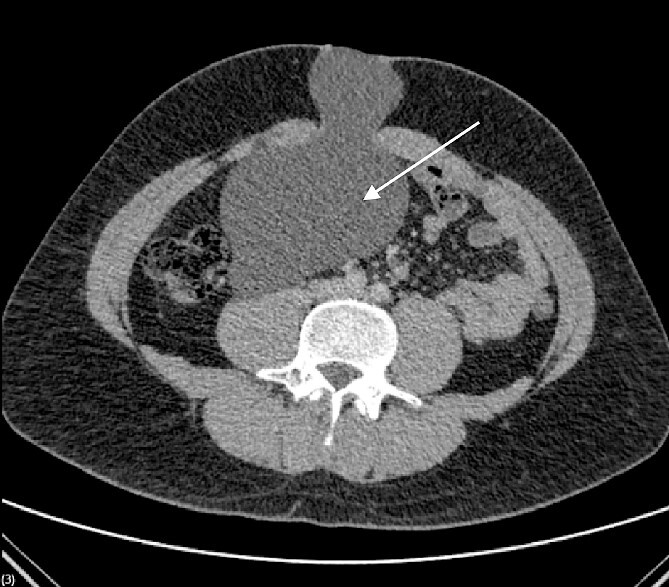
CT scan demonstrating bilobed cystic mass.
